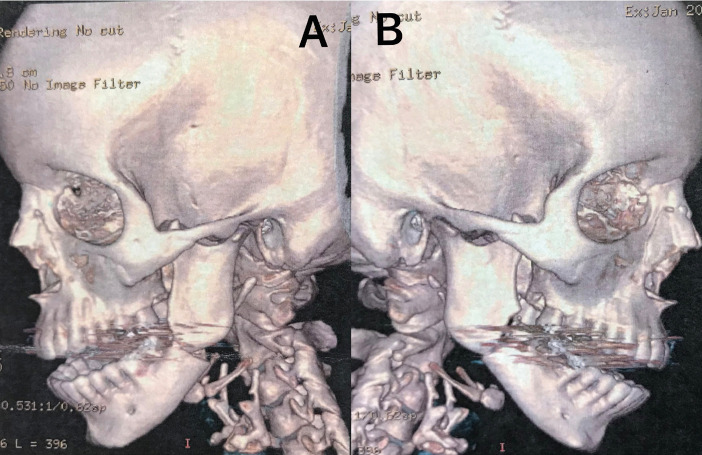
Computed tomogram in 3D reconstruction demonstrating fracture of angle involving the post-extraction area. A: Fracture of left angle. B: Fracture of right angle.
